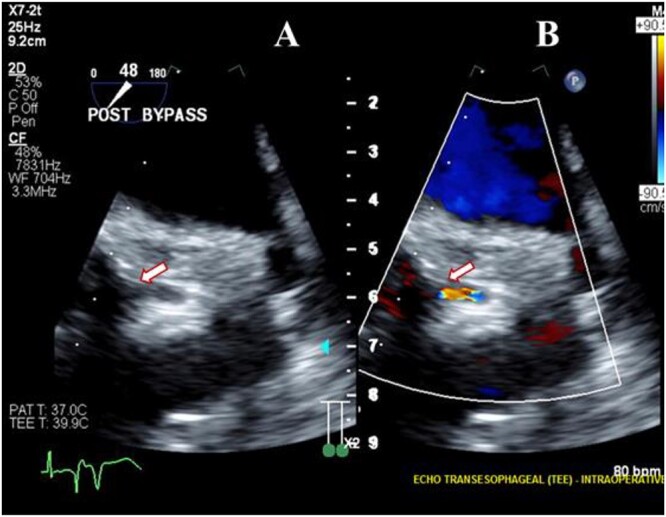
Transesophageal image following re-operative AVR showing severe stenosis (arrow) at the origin of the left main coronary artery (A) with turbulent flow (arrow) on color Doppler imaging (B).
